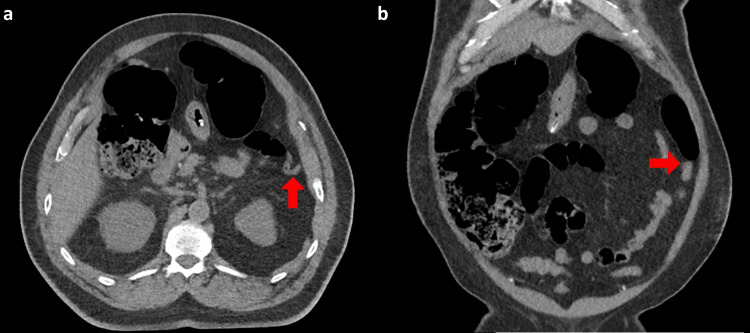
Non-contrast computed tomography scans on admission. The images show (a) axial slice demonstrating the abrupt caliber change at the splenic flexure (red arrow) and (b) coronal slice showing dilated right colon with abrupt transition to collapsed sigmoid (red arrow).
